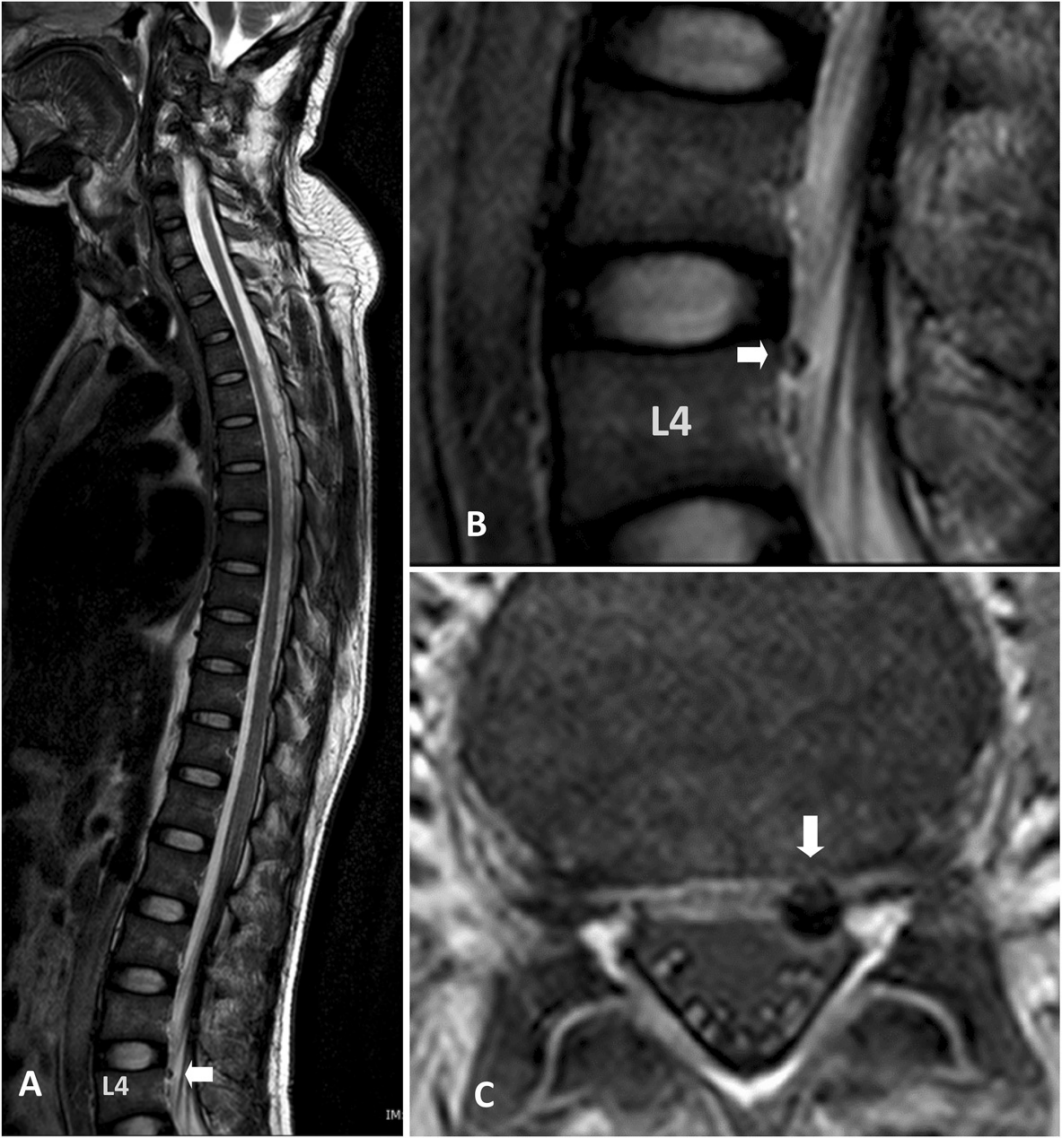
Magnetic resonance imaging scan of the cervical spine taken one day after stem cell transplantation via lumbar puncture technique. A sagittal T2-weighted scan of his whole spine (A, B) and an axial T2-star scan of his lumbar spine (C) shows hyposignal intensity of paramagnetic substance tagged stem cells in the subarachnoid space of the lumbar spine and some in the cauda equina. No demonstrable paramagnetic tagged stem cell was seen at the cervical spine level. (White arrow; hyposignal intensity in the subarachnoid space at lumbar spine).
